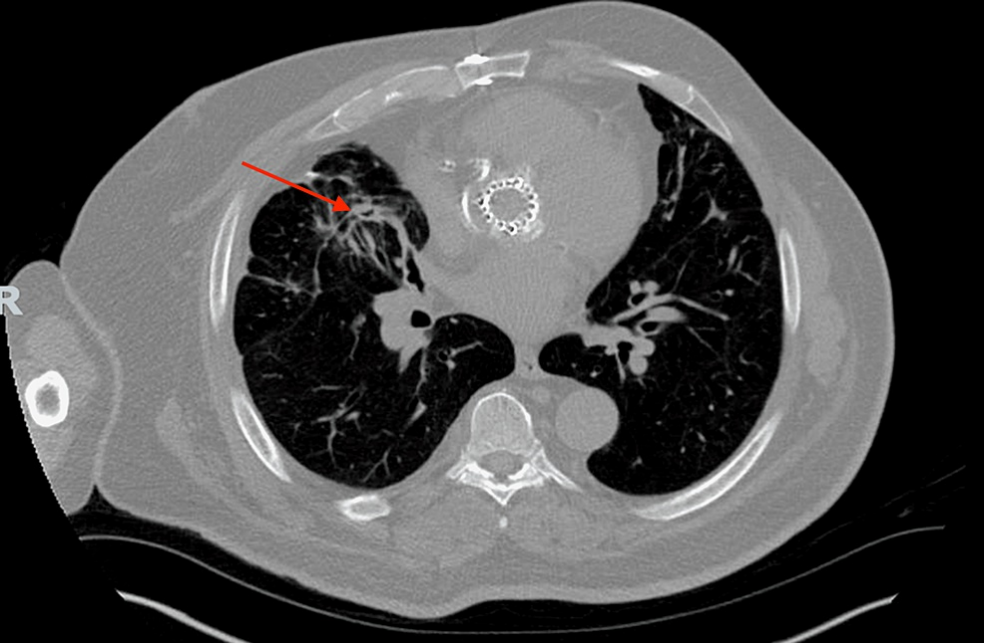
Post-operative CT imaging following right lower lung wedge resection Axial CT image showcasing the thoracic region after the patient underwent a right lower lobe wedge resection.
